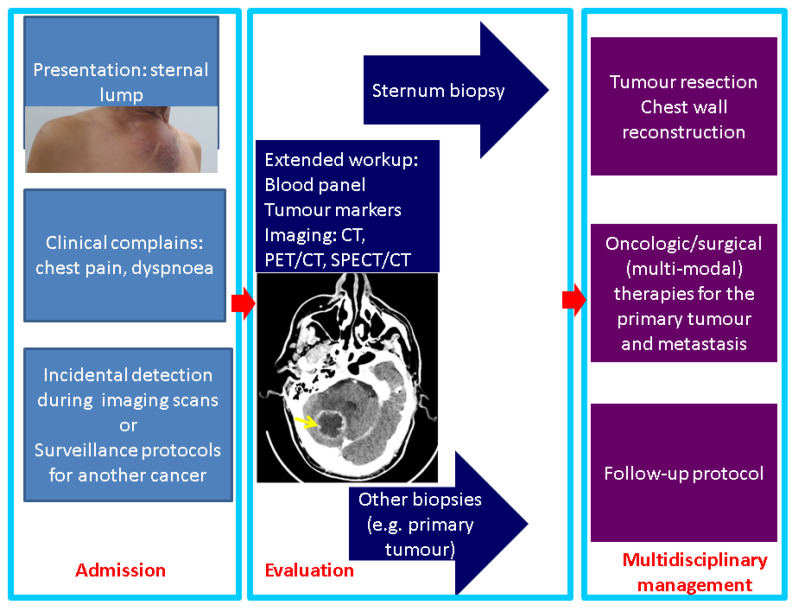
Panel of management in sternal metastases: from presentation to therapy (the photo of the sternal lump represents the case previously described in a 61-year-old male who was admitted for the first time for this breastbone mass, which was finally confirmed as metastatic lung cancer; the image in the second box represents a CT scan with brain metastasis in the same patient).
